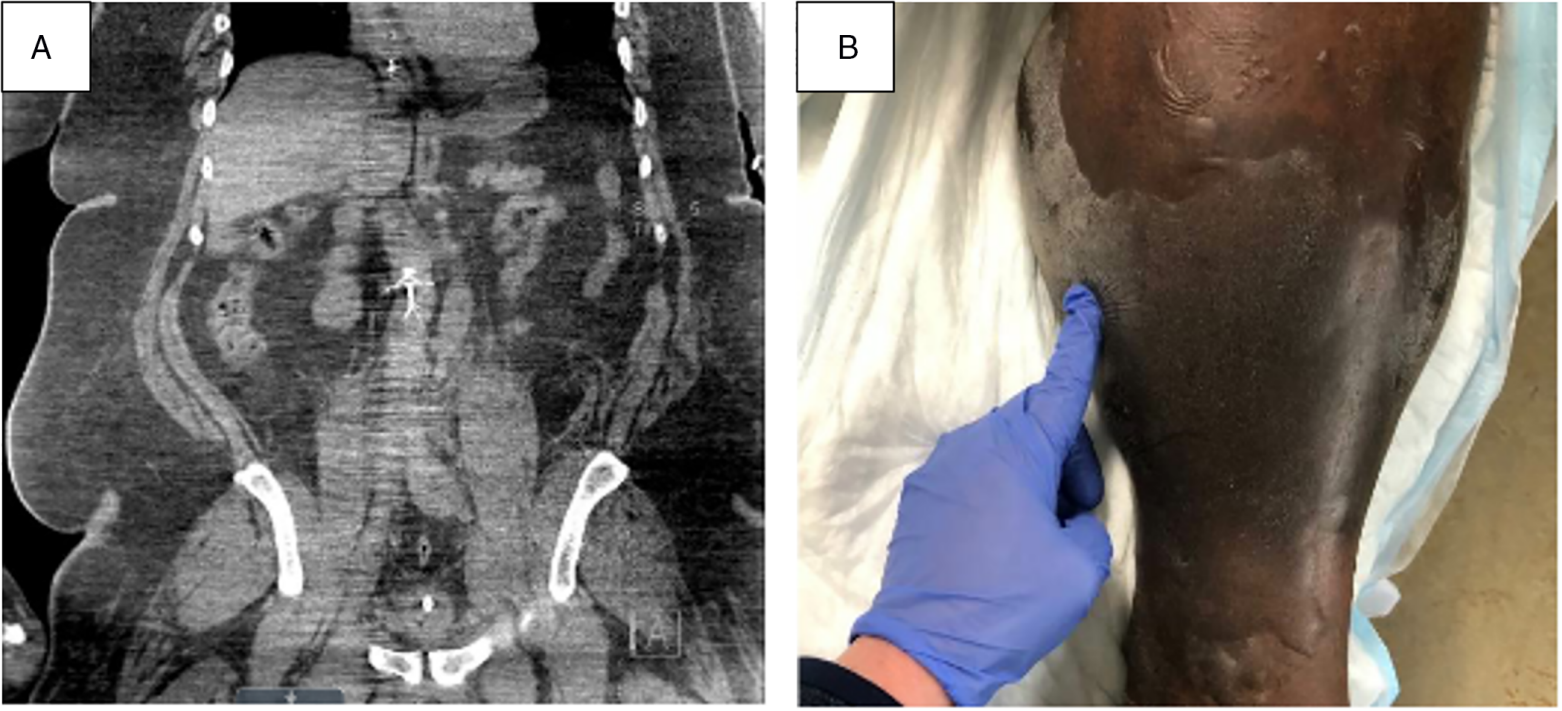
(A) Coronal view of the computed tomography imaging of the abdomen and pelvis shows dilation of the distal inferior vena cava below the level of the inferior vena cava filter. (B) Tense lower extremity oedema with discolouration and fluid‐filled bullae.
